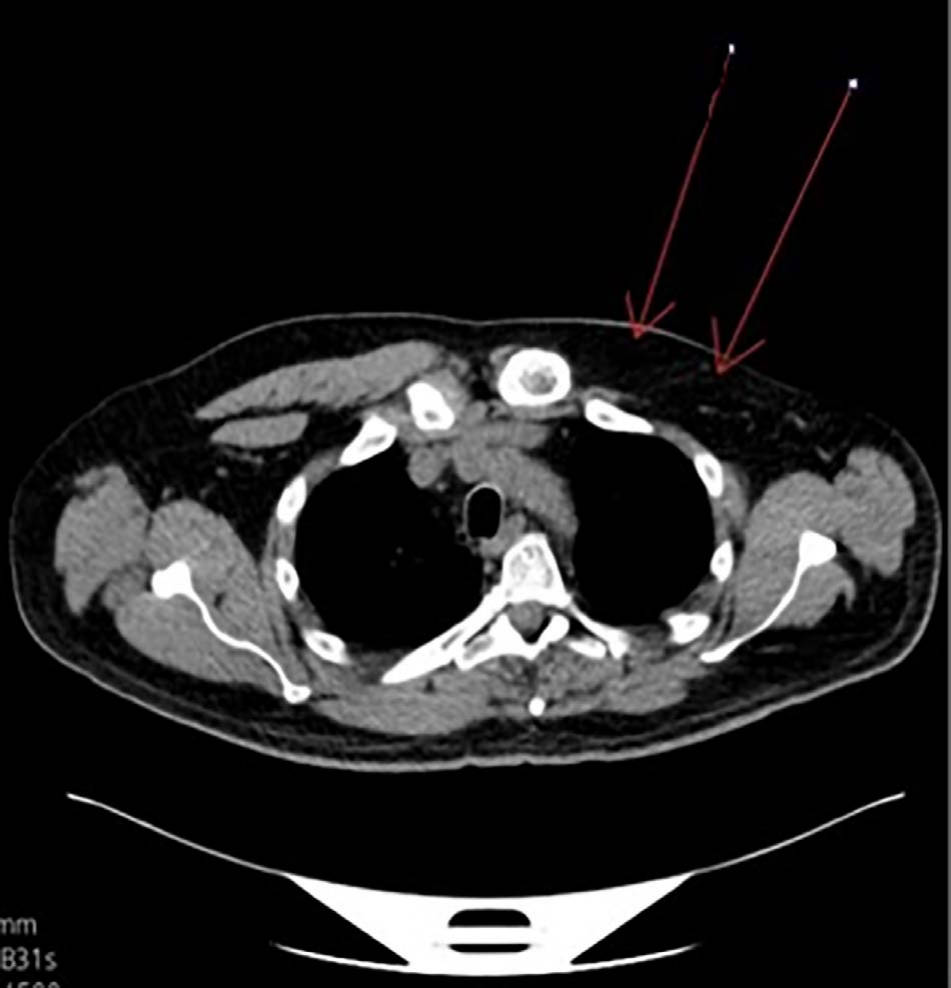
Noncontrast transaxial computed tomography scan shows the absence of the left pectoralis major and pectoralis minor muscles (arrows).
